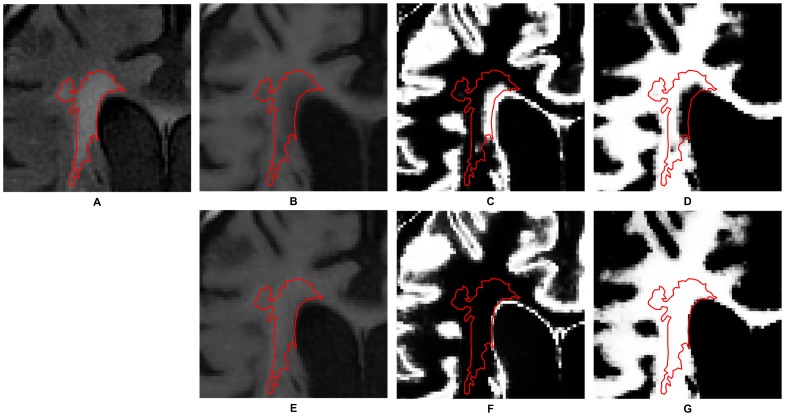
The effect of fusing T1w and FLAIR images. The red contour in these images represents the position of WMHs delineated semi-automatically by the experienced neurologist. (A) An FLAIR image. (B) The registered T1w image. (C) The probabilistic map representing the gray matter segmented from B. (D) The probabilistic map representing the white matter segmented from B. (E) The registered T1w image after being fused with the FLAIR image. (F) The probabilistic map representing the gray matter segmented from E. (G) The probabilistic map representing the white matter segmented from E. Note that in the probability maps, higher intensity corresponds to higher probability.
